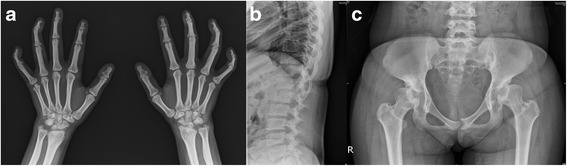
Radiographs of 19-year-old Korean female with ML III. a Radiograph of hands and wrists showing claw hands: impossible flexion and maximum extension without objective skin thickening or joint involvement. Small and irregular carpal bones and wide proximal phalanges are noted. b Radiograph of spine showing irregularity of the vertebral end plates. c Radiograph of pelvis showing flattened acetabulum and femoral head deformity
